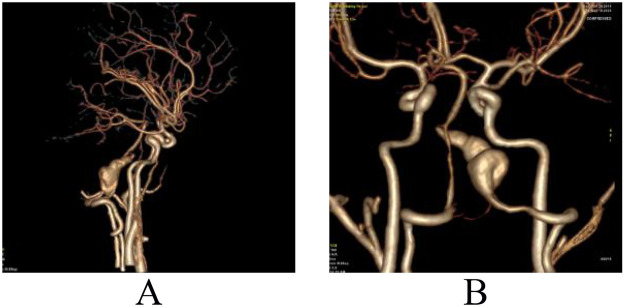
(A,B) 3D CTA reconstruction reveals irregular dilatation and wall thickening of the right vertebral artery (V4 segment), extending approximately 35 mm in length.
